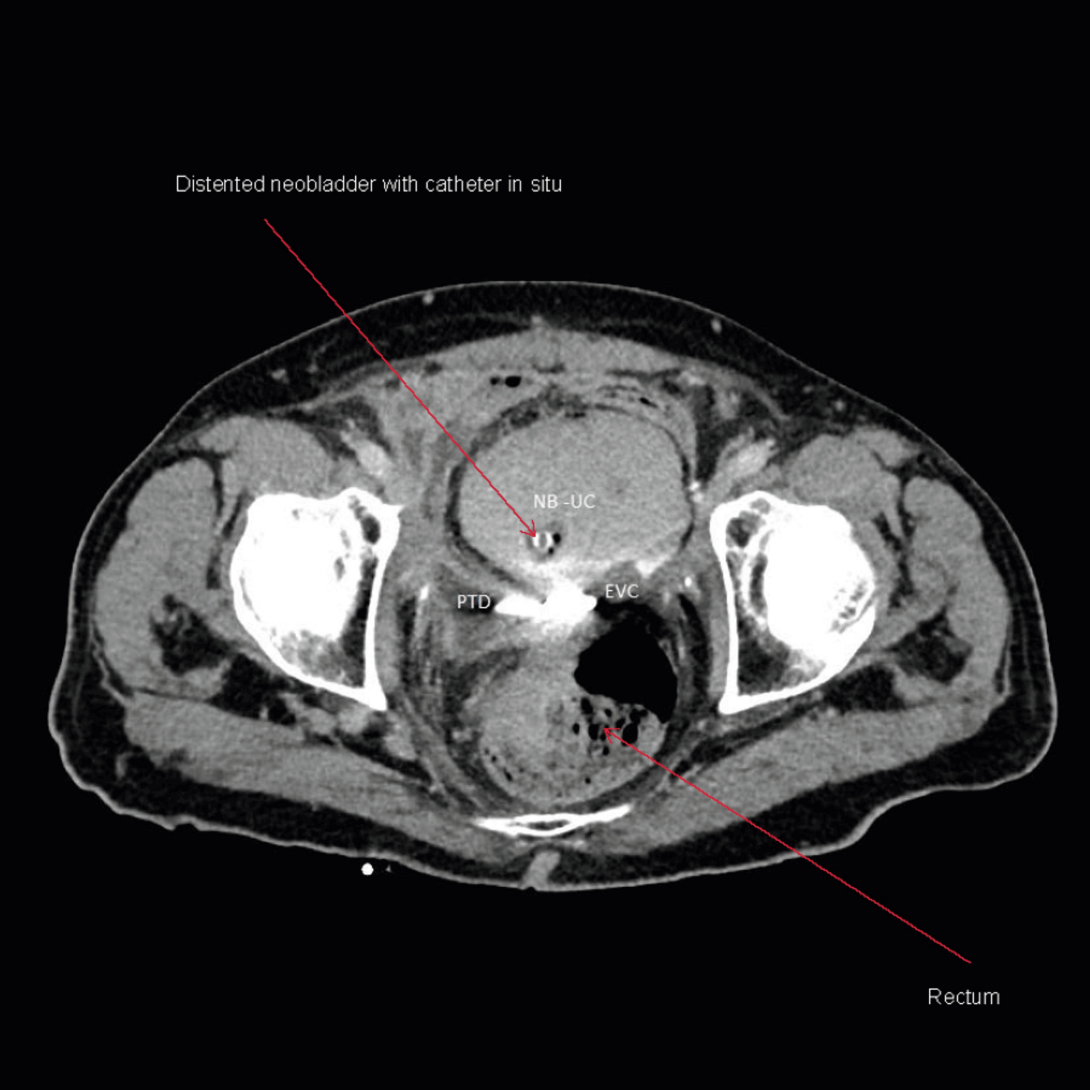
Axial view of CT cystogram two weeks post-admission and conservative management showing neobladder with urethral catheter in situ and minimal extravasation of contrast from the neobladder neck. Internal coiled end of the pigtail drain is visible as hyperdense shadow behind the neobladder. There is near complete resolution of pelvic collection NB-UC: Neobladder urethral catheter; PTD: internal end of pigtail drain; EVC: extravasation of contrast
